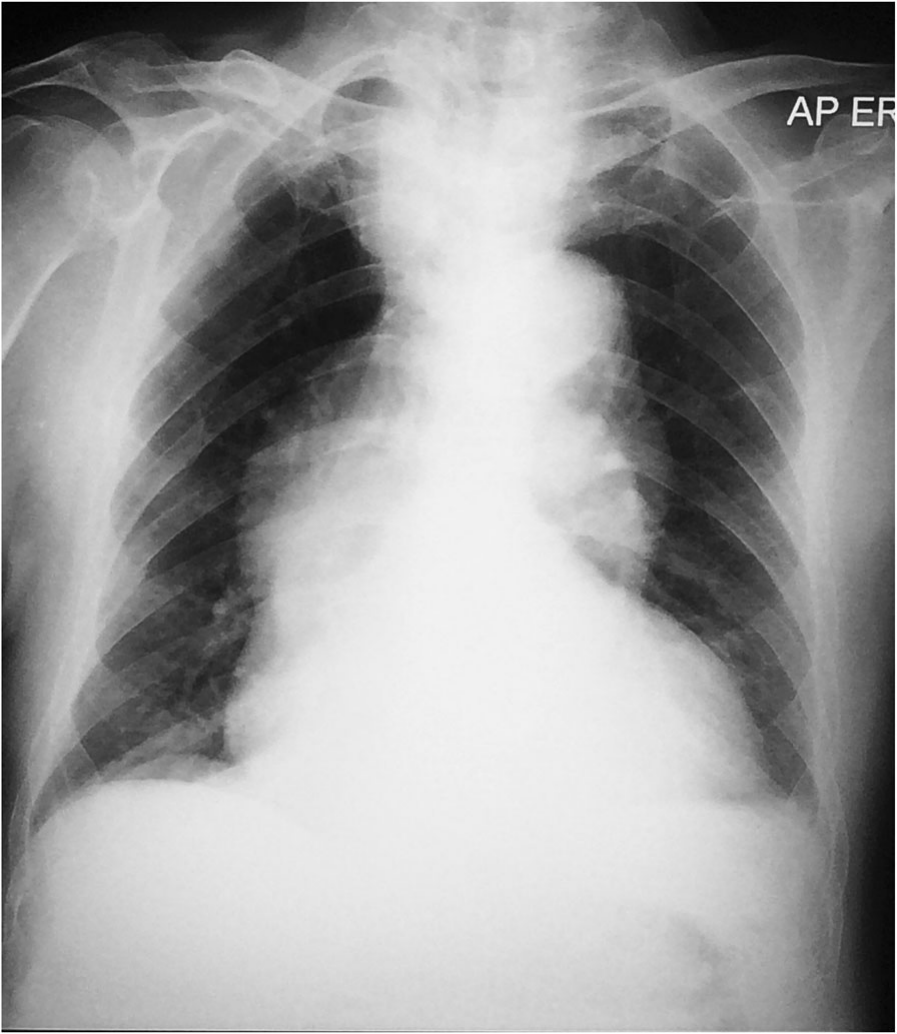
Chest radiograph (anteroposterior view) shows prominent hilar opacities
